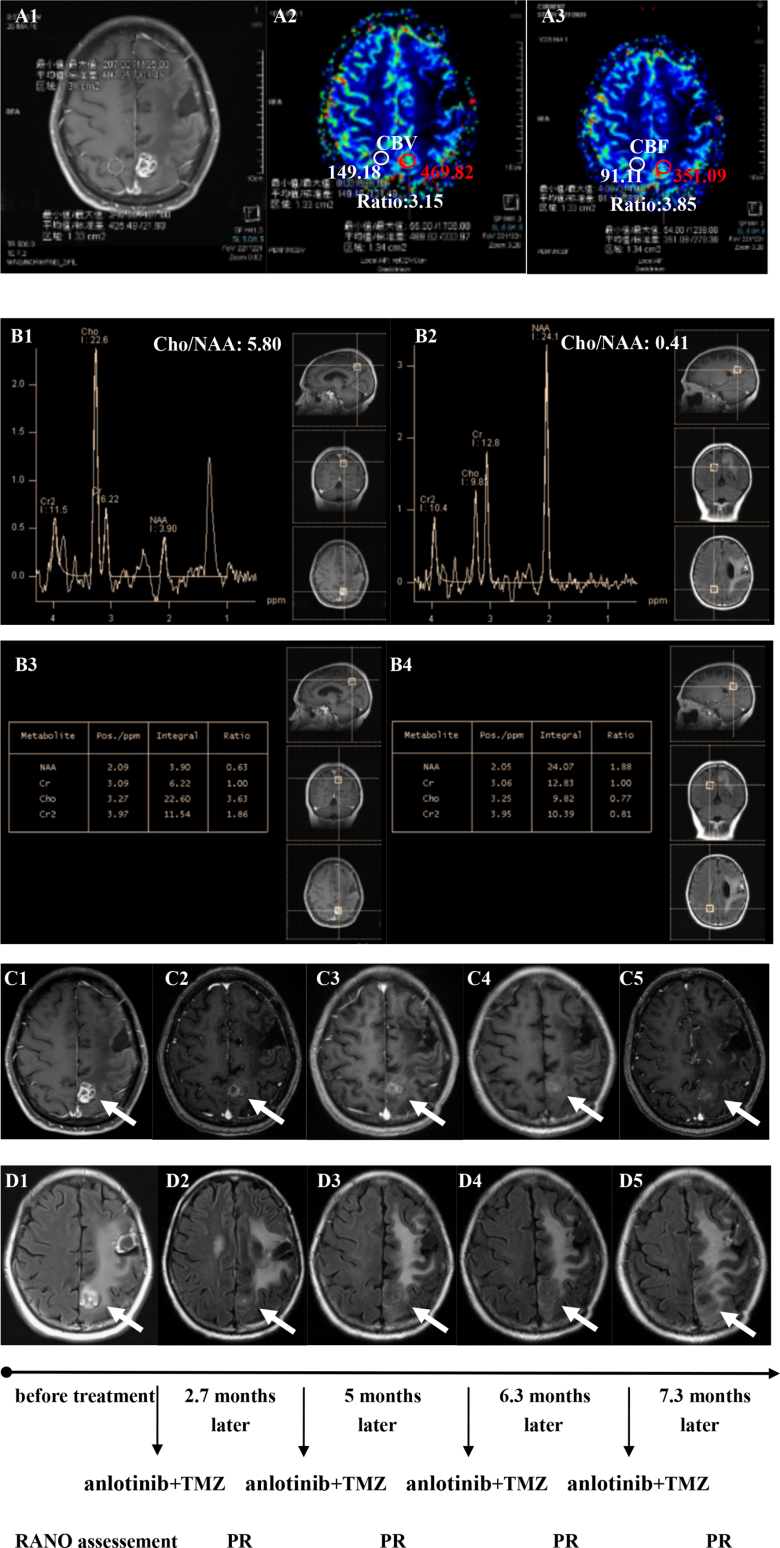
Brain scan of patient number 17 (IDH1−; Recurrence occurred at a second site; The interval time between the end of CCRT and the treatment of anlotinib combined with TMZ was 2.9 months). (A1–A3) Perfusions maps at the time of recurrence; (B1–B4) MRS at the time of recurrence; (C1–C5) Contrast-enhanced MRI; (D1–D5) MRI-Flair; MRI images followed-up every 1 to 3 months before and after treatment. The arrow refers to the area of recurrence. The patient achieved partial remission after treatment and had a progression-free survival time of 8.8 months.
